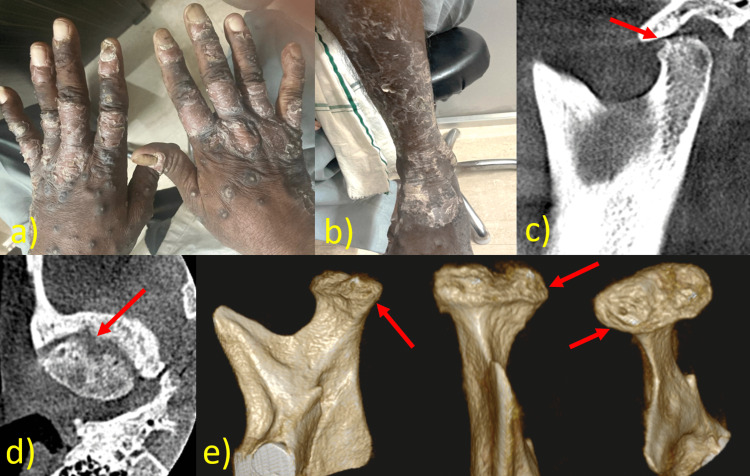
a) Presence of psoriatic patch over hands and feet, b) oblique sagittal section shows concave-shaped remodeling with multiple osteophytes of the superior surface, c) oblique axial section shows an osteophyte in the superior and anterior surfaces along with erosion. d) 3D CBCT CBCT: cone beam computed tomography
